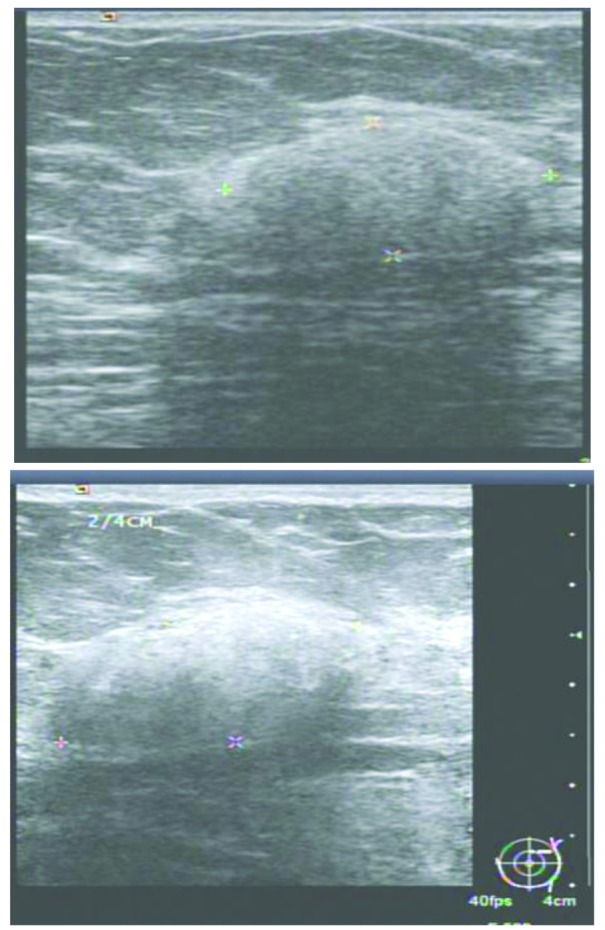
Sonography revealing a 2–3-cm, well-encapsulated mass in the left breast with complex scattered echogenic areas measuring ~2.42×2.17×1.36 cm, which displaced the adjacent normal breast tissue and was consistent with a diagnosis of lipoma and internal inhomogeneity.
